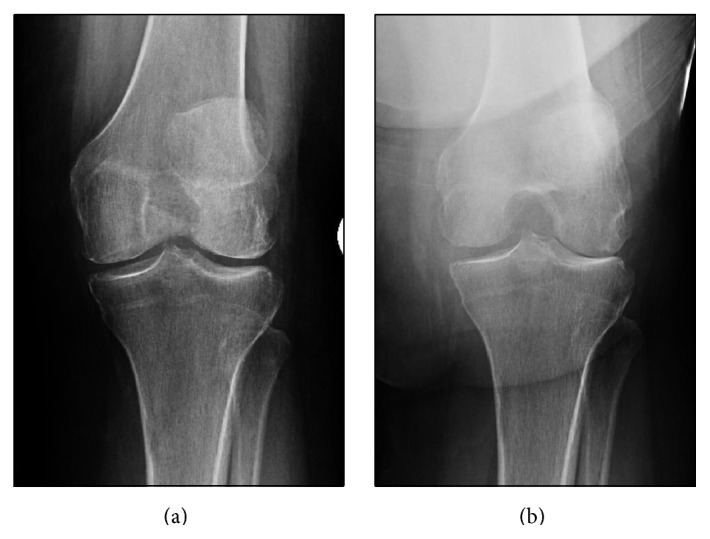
AP radiograph of a left knee (a). The tunnel view shows lateral compartment joint space narrowing (b).
